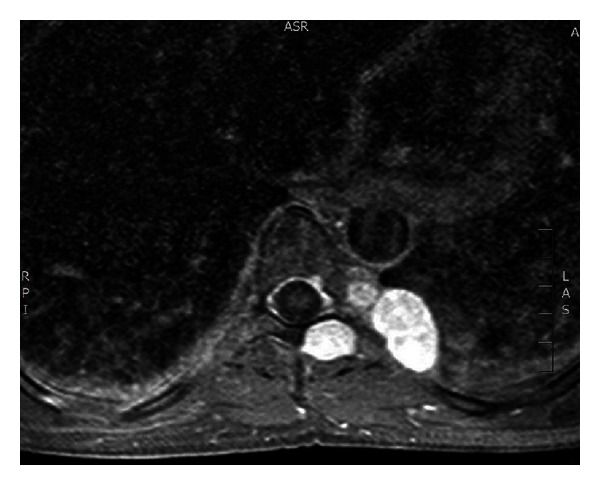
Magnetic resonance imaging (MRI) showing multiple paravertebral schwannomas.
